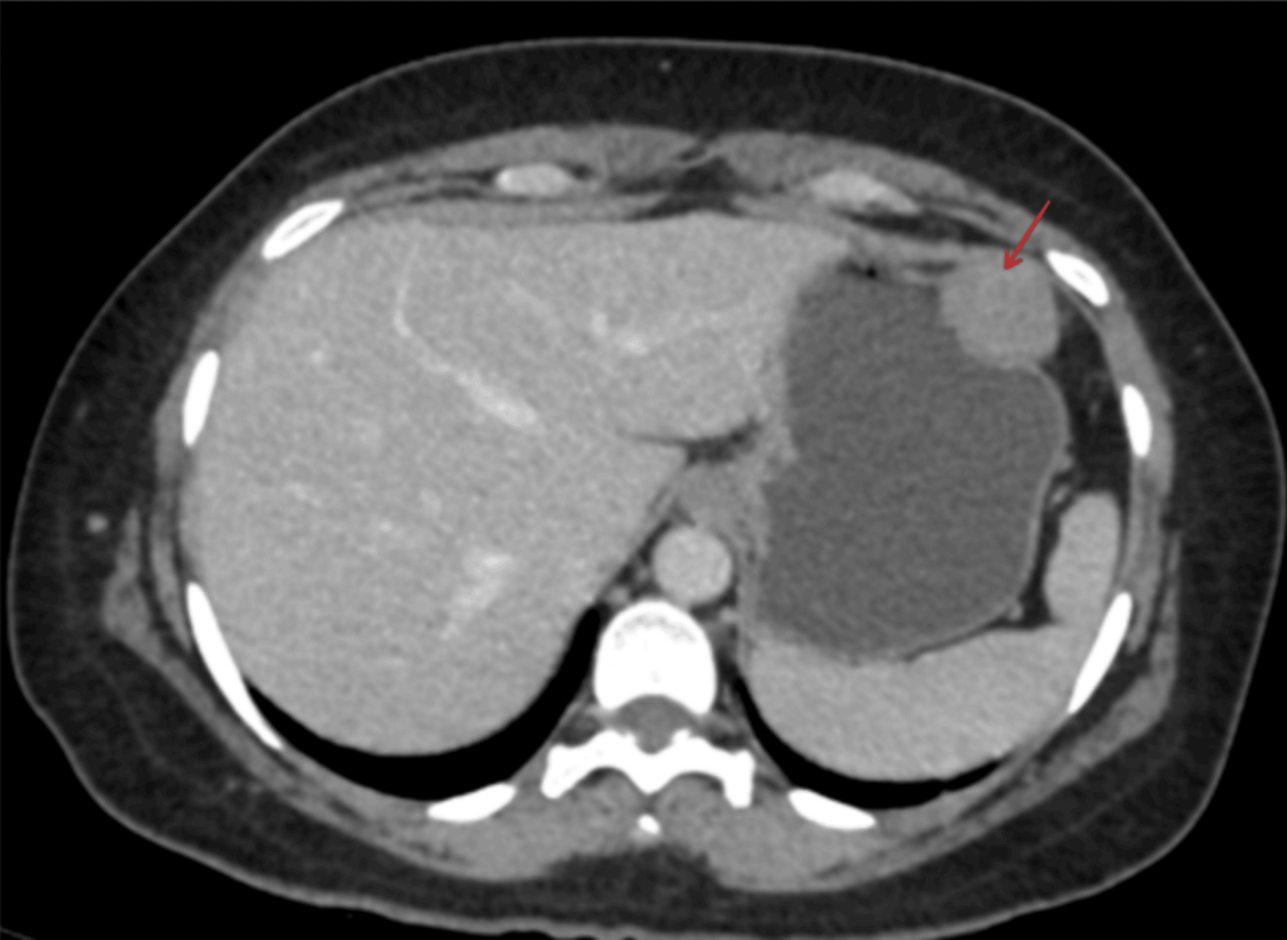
CECT findings of the abdomen In the CECT scan, the red arrow indicates the extraluminal mass originating from the greater curvature of the stomach. CECT, contrast-enhanced CT
